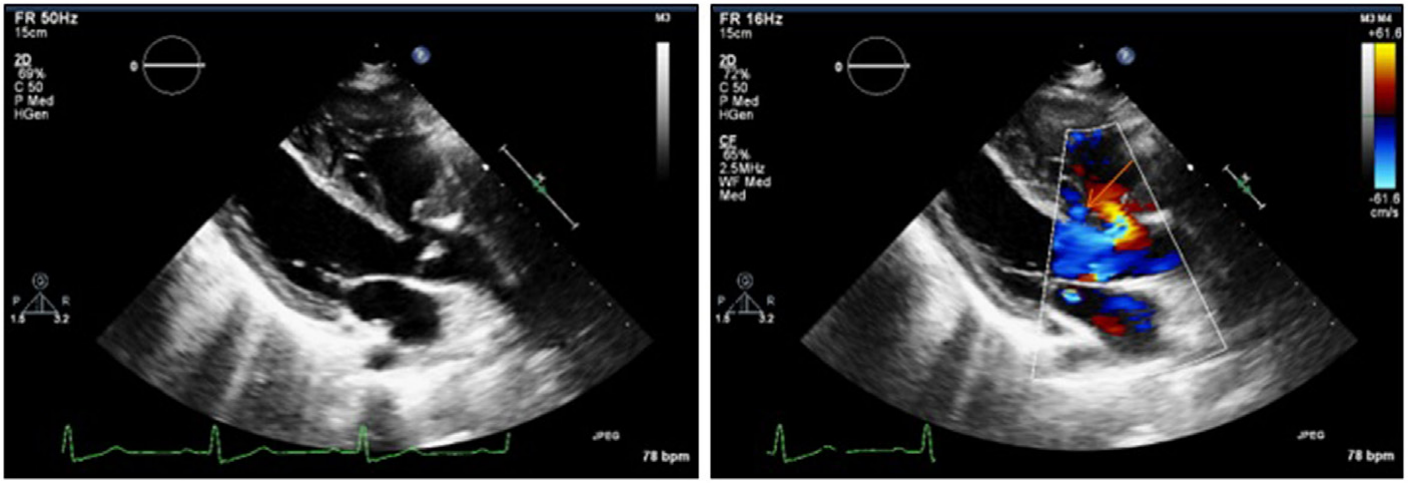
Preoperative transthoracic echocardiogram showing a membranous ventricular septal defect with predominant right to left shunting (see orange arrow).
